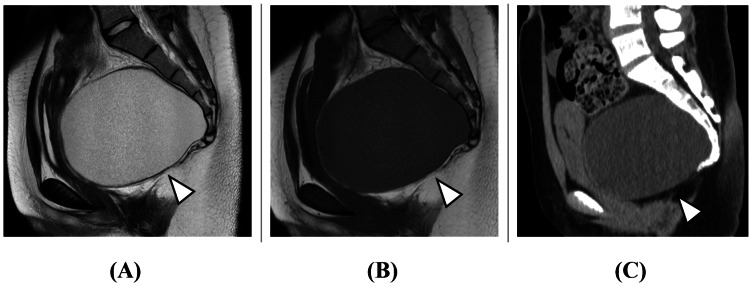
Initial pelvic imaging. (A) MRI T2 sagittal. (B) MRI T1 sagittal. (C) CT sagittal. A 12 cm cystic mass was observed posterior to the rectum, with homogeneous MRI T2 high intensity (arrowhead in Figure 1A), MRI T1 low intensity (arrowhead in Figure 1B), and CT iso density (arrowhead in Figure 1C) fluid content. The cyst wall was thin and uniform without apparent nodular lesions, suggesting against malignancy.
